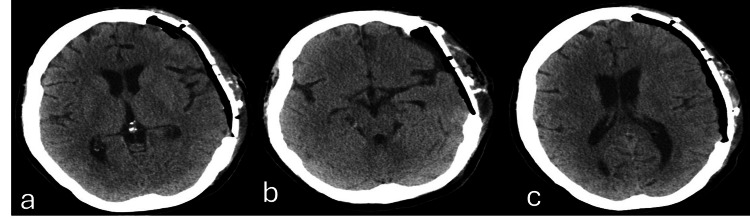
Axial plane of brain computed tomography obtained after cranioplasty Closure of the left fronto-temporo-parietal craniectomy area with cranioplasty plate – PEEK (a). Underlying the PEEK prosthesis there is a thin epidural collection with air component, without significant hemorrhagic component or a significant mass effect on the endocranial structures (b, c). PEEK, polyetheretherketone
